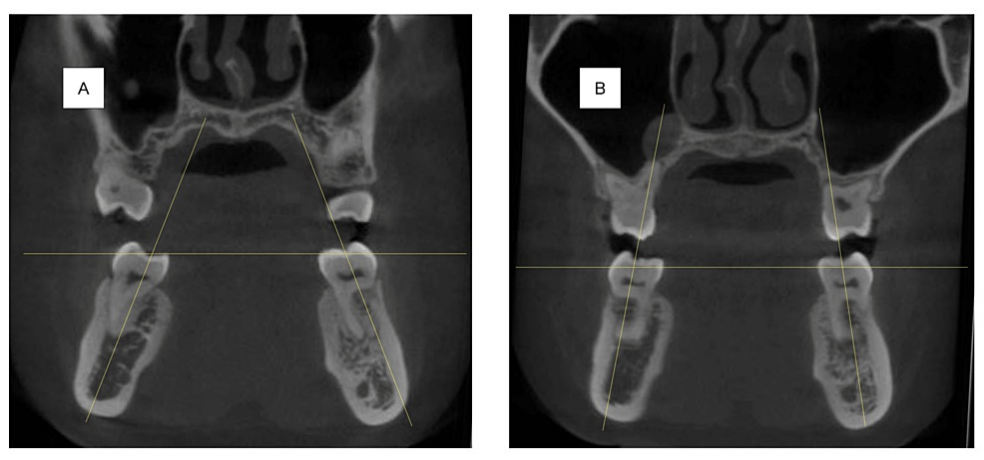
Coronal view of CBCT scan showing angular measurements of mandibular first molars (A) Preoperative CBCT image showing the coronal section of mandibular first molars. (B) Postoperative CBCT image showing the coronal section of mandibular first molars.
